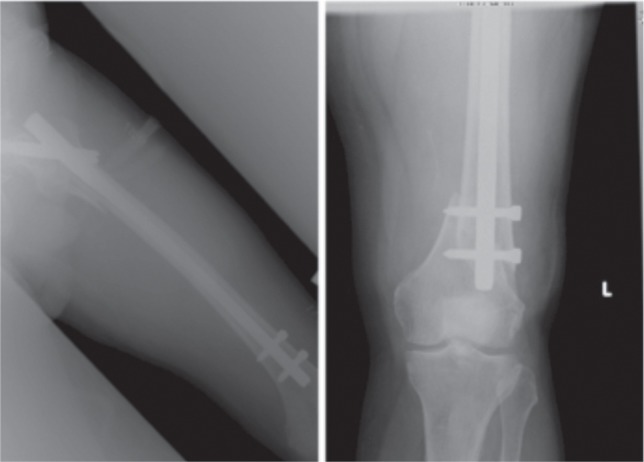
(i) Radiograph left femur after Gamma nail insertion. (ii) Radiograph left distal femur after 2nd admission.
